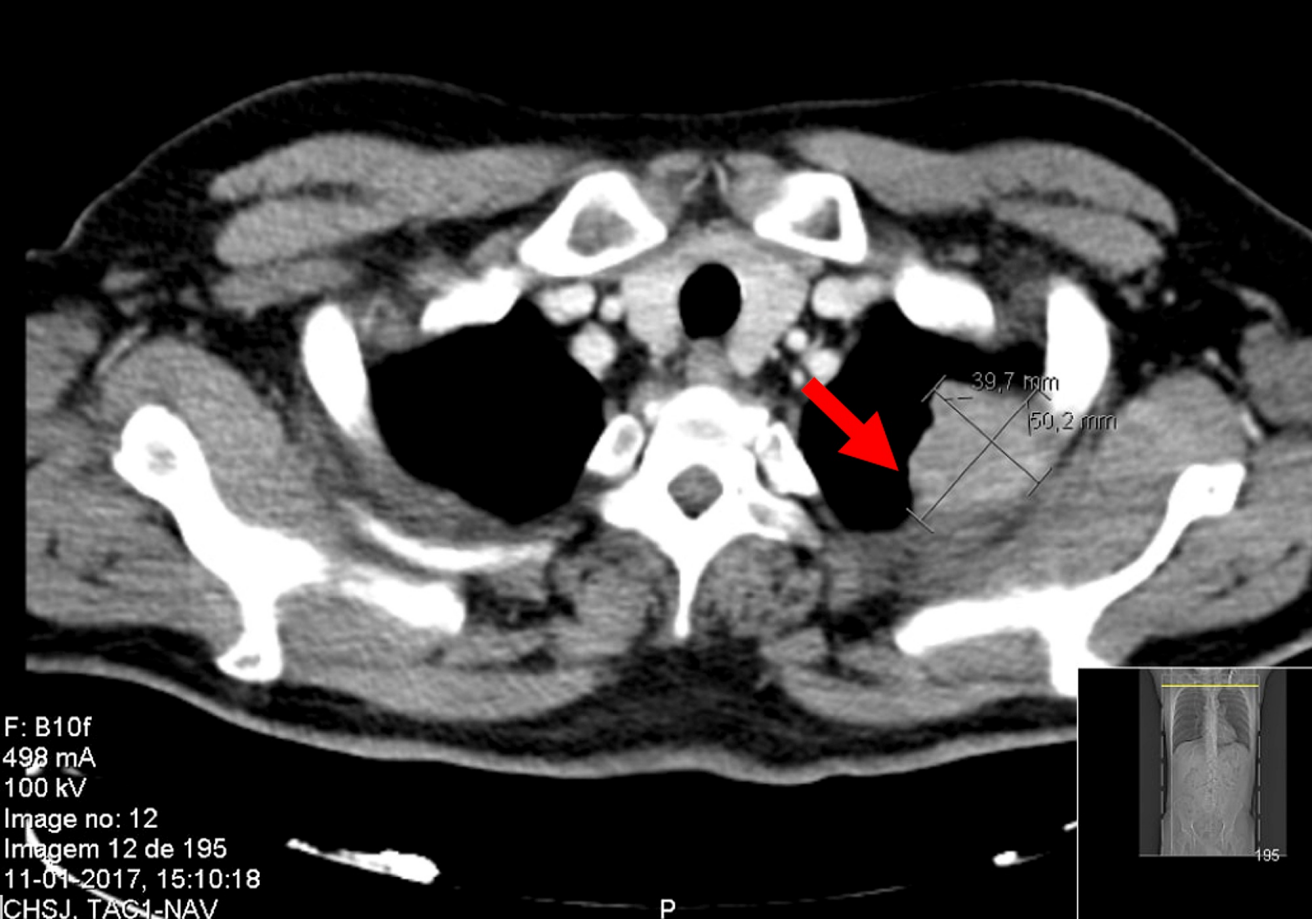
CT scan revealing an upper left lung mass of 40x50 mm, with the invasion of soft tissues within the second intercostal space
